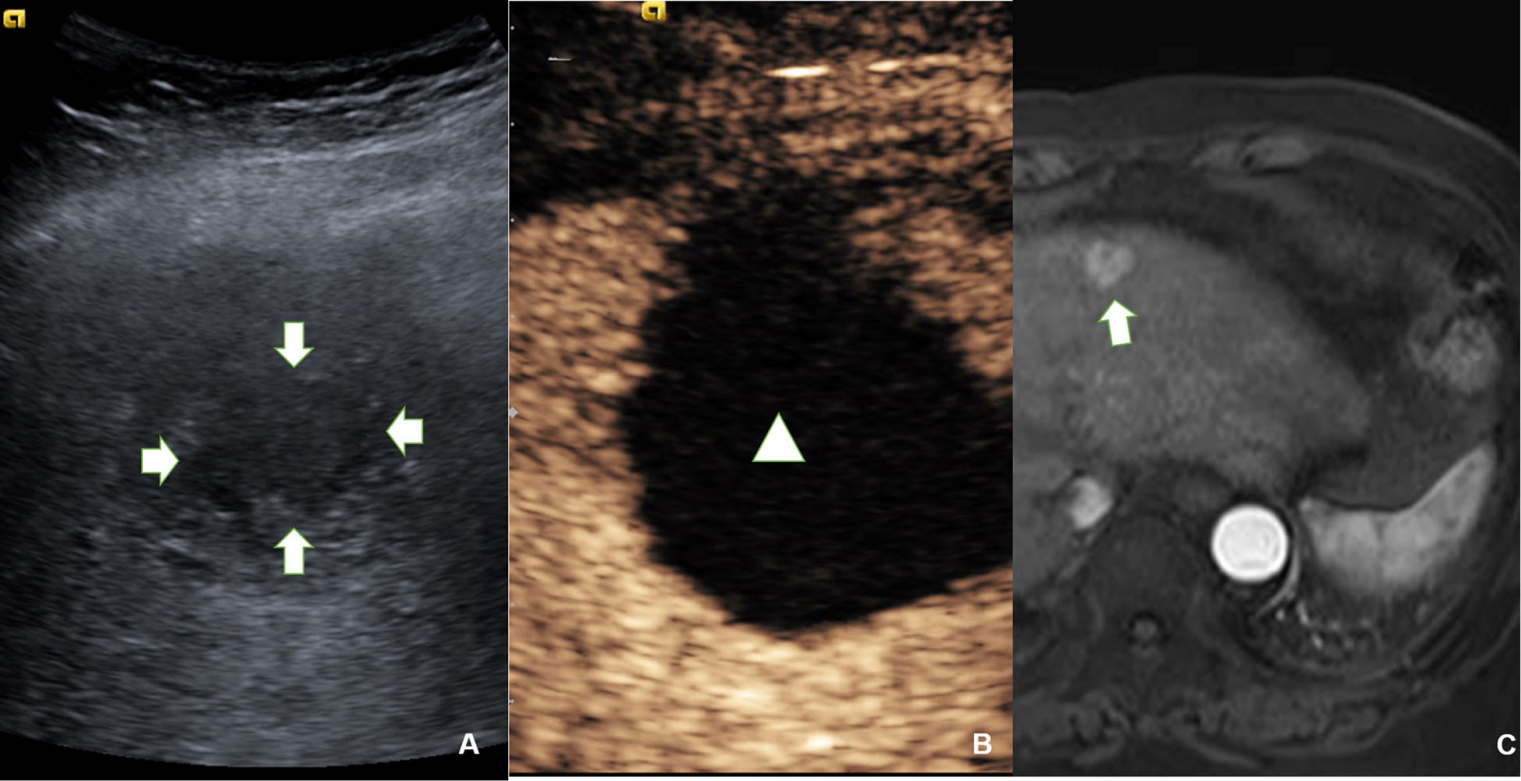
Relationship between a larger dimension of non-enhancing area in preoperative SMA-CEUS and tumour response after TACE. A, B-mode ultrasound showed a hypoechoic HCC lesion located in segment 4, which was 3.3 × 3.5 cm (indicated by the arrow). B, Preoperative SMA-CEUS showed that a significant larger dimension (3.9 × 4.6 cm) of non-enhancing area (triangle shown) than the size in B-mode ultrasound. C, Postoperative abdominal contrast-enhanced MRI showed that the tumour response should be defined as stable disease (indicated by the arrow). TACE = transarterial chemoembolization, HCC = hepatocellular carcinoma; CEUS = contrast-enhanced ultrasound; SMA-CEUS = superior mesenteric artery contrast-enhanced ultrasound
